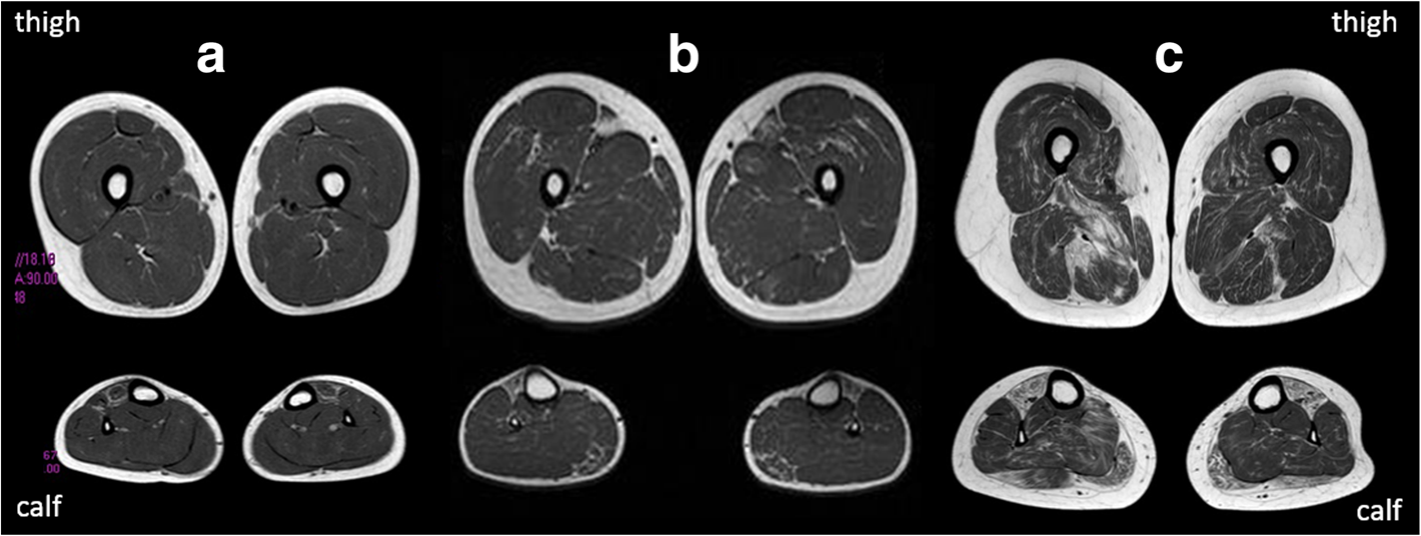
Muscle MRI of lower limb of three cases with different severity. Upper images refer to thigh, whereas lower images refer to calf muscles. Case 7 in (a) is the less affected and only initial fat substitution of tibialis anterior is noted. This feature is shared by all the patients. Interesting, in this case only, the tibialis involvement seems to start from the periphery of the muscle, a feature which has been already described in quadriceps muscles of COL6 mutated patients. In (b) involvement of medial gastrocnemius and very initial involvement of vastus medialis is present. This MRI belongs to case 6 at age 16. In (c) the tibialis anterior is totally replaced by fat tissue. Medial and lateral gastrocnemii are also affected. In the thight, vastus medialis and adductor magnus are involved but rectus femori is spared. This is case 5 and MRI was performed at 44 years of age
